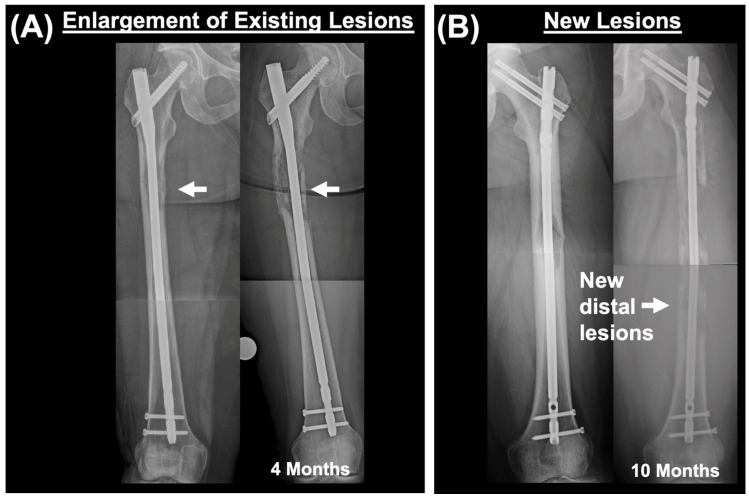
Categorization of cancer progression. (A) Immediate post-nailing femur and subsequent cancer progression (4 months post-op). Cancer progression categorized as enlargement of osteolytic lesion that was already present at time of intramedullary nail placement. Arrow shows site of osteolytic lesion. (B) New lesions identified on follow-up X-rays after intramedullary nailing. New lesions are distal to the most distal lesion that was identified at time of nailing. Time difference between two X-rays is 10 months.
